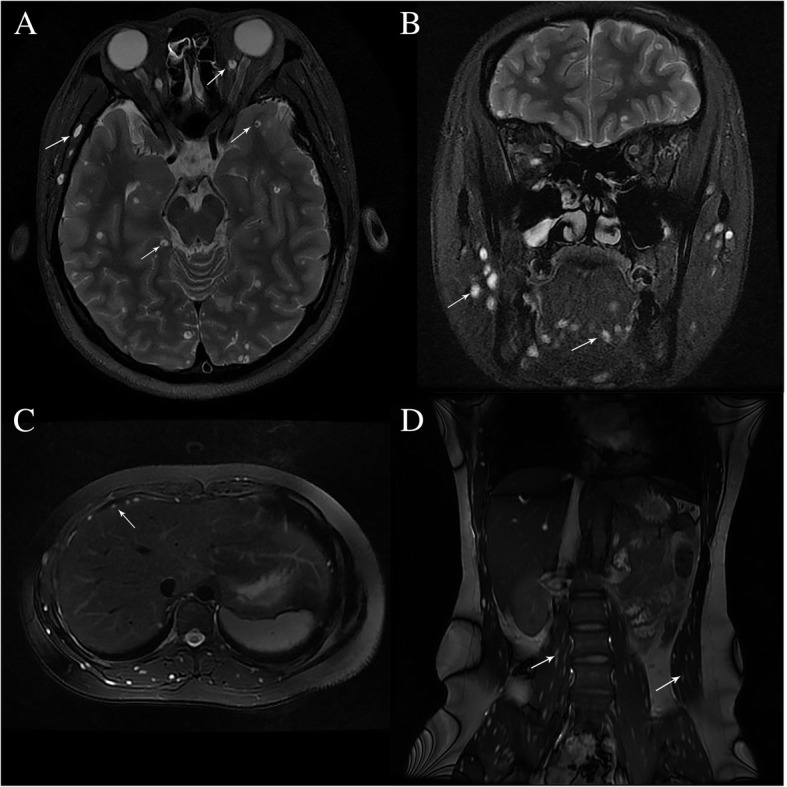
MRI of the brain, orbit, and body. Fat-suppressed T2-weighted axial and coronal images of brain and abdomen showed numerous well-defined hyperintense lesions with eccentric scolex involving the (a) left temporal lobe, left orbital muscle, right diaphragm, right occipital lobe, (b) masseter, tongue muscles, (c) erector spinae, and (d) psoas magnus muscles
